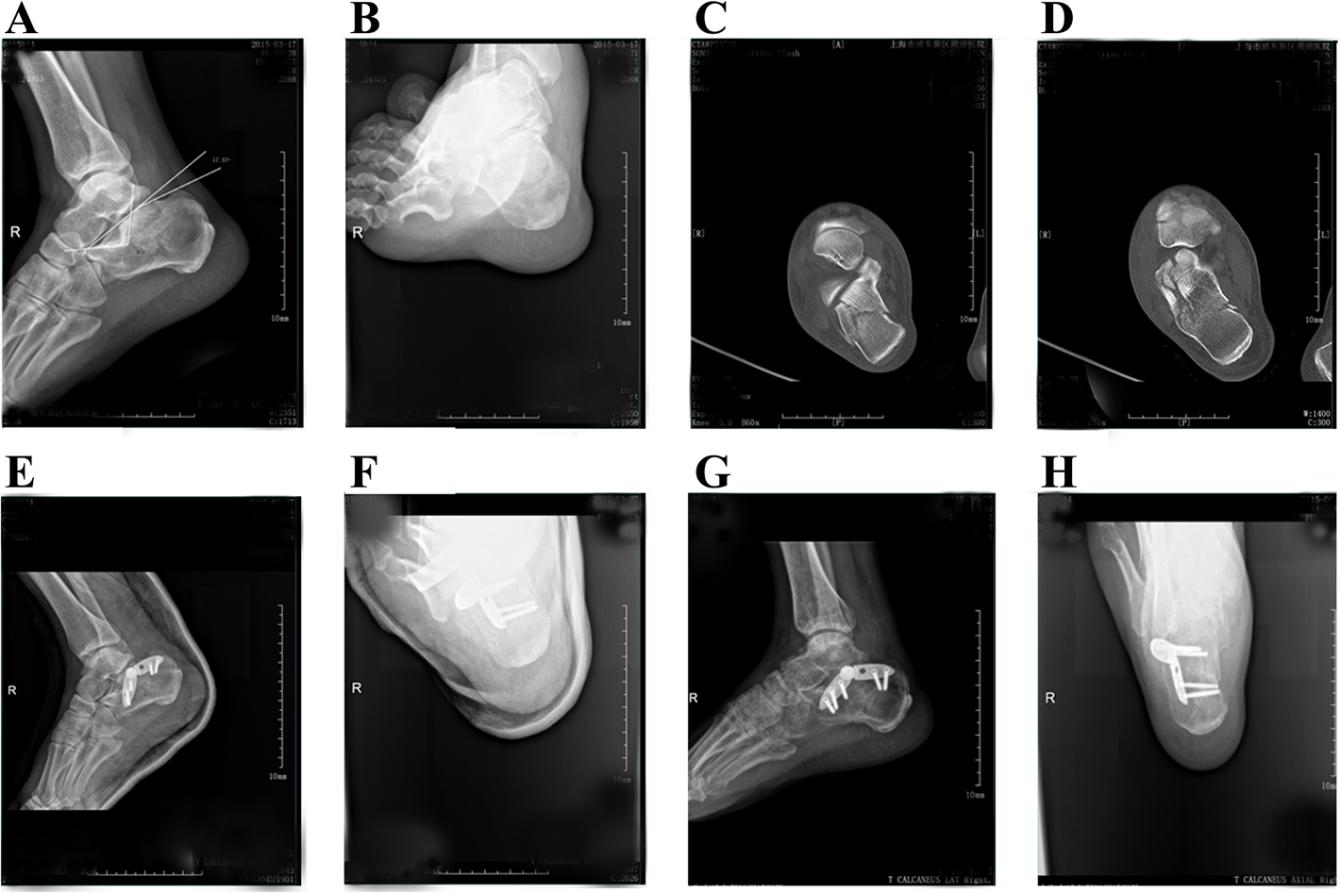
A 41-year-old male patient was admitted to our hospital because of pain and swelling on the right foot after falling from a height. Preoperative lateral (a) and axial (b) radiographs showing the right calcaneal fracture, with reduced Böhler angle (17.37°) and Gissane angle (97°). Preoperative horizontal (c) and coronal (d) computed tomography images showing a Sanders IIA fracture with a bulked lateral wall. Postoperative lateral (e) and axial (f) radiographs showing obvious corrections of the Böhler angle (23.17°) and Gissane angle (124.4°). Postoperative lateral (g) and axial (h) radiographs showing excellent fracture union, with a Maryland foot score of 87
